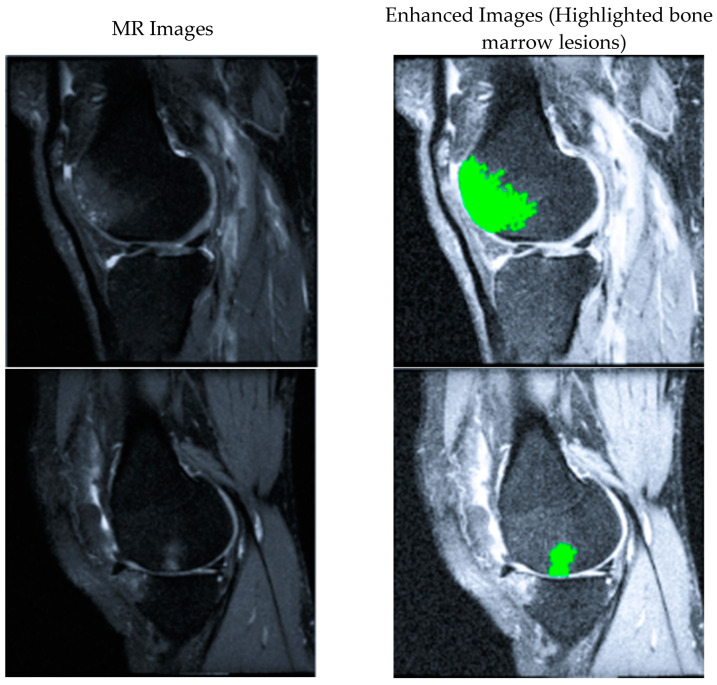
Two examples of MR images with bone marrow lesions (BMLs) marked in green.
